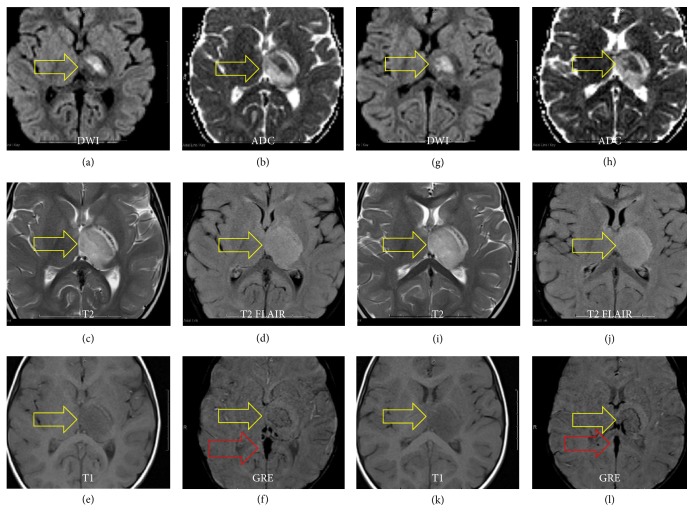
MRI obtained to confirm deep cerebral venous thrombosis and left thalamic infarction. DWI and ADC imaging ((a), (b), (g), and (h)) shows restricted diffusion in the anteromedial left thalamus without distinct territorial boundaries in relation to venous ischemia. T2 FLAIR hyperintensity ((c), (d), (i), and (j)) shows surrounding edema related to the infarction. GRE image (f) shows dark signal loss suggestive of petechial hemorrhage around the area of ischemia. GRE image (l) shows linear signal drop in the posteromedial surface of the left thalamus, highly suggestive of thrombus within a venous structure, likely part of the left internal cerebral vein.
